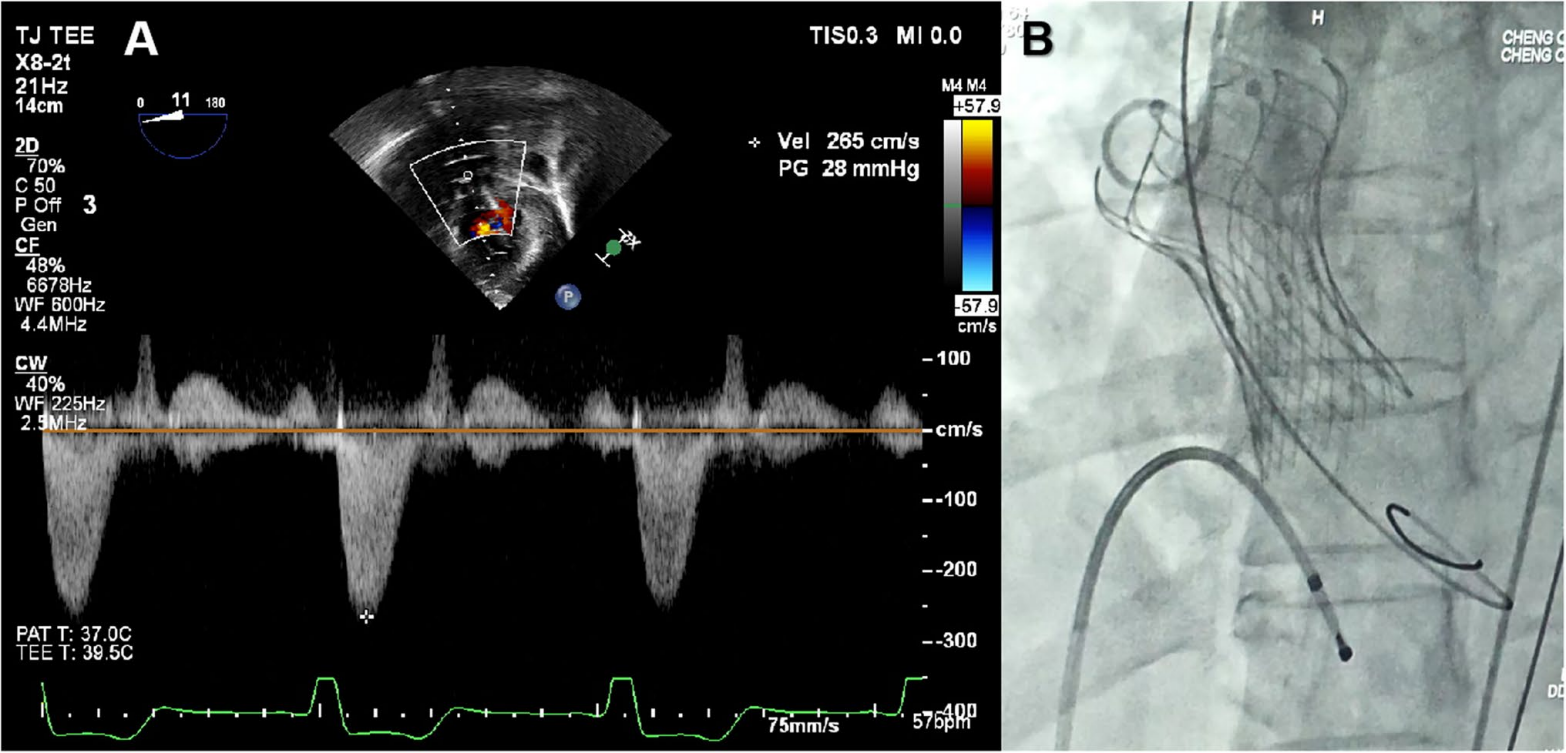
After TAVI, the transesophageal echocardiographic image demonstrated the successfully implanted transcatheter aortic valve with a mean transvalvular pressure gradient of 28 mmHg. The corresponding digital subtraction angiography (DSA) confirmed satisfactory valve deployment and preserved coronary perfusion
